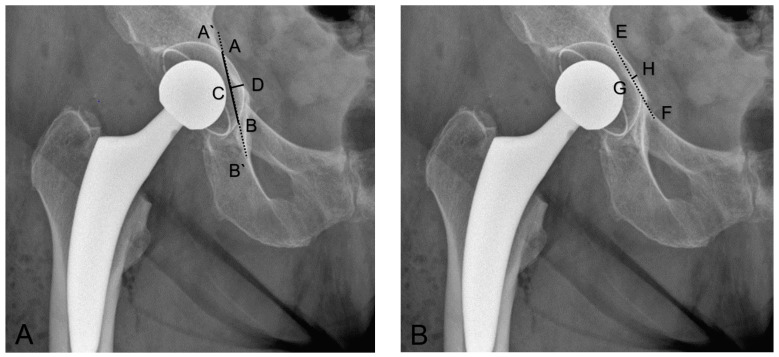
Radiographic measurements of the medial cup protrusion. (A). AB length of the overlap tangent was defined as the distance between the two crossings of A′B′ the ilioischial line and the cup; CD ilioischial overlap as the distance between the ilioischial line and the tangent of the acetabular cup medial. (B). GH iliopectineal distance as the minimal distance between the iliopectineal line (EF) and the cup [4].
